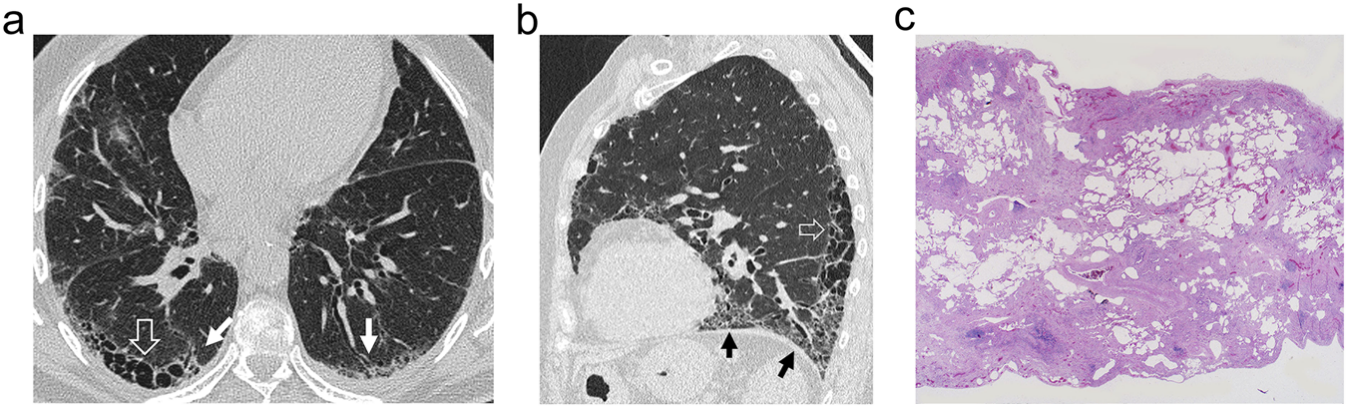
UIP. a CT shows subpleural cystic lesions in the right upper lobe (open arrow) and bilateral traction bronchiectasis (white arrows). b Distribution of abnormalities are shown in the sagittal section of the left lung, with involvement of the most basal lung next to the diaphragm (arrow), with cysts in the middle posterior zone. c Surgical lung biopsy of the left lower lobe demonstrated a UIP pattern with patchy, heterogeneous fibrosis
